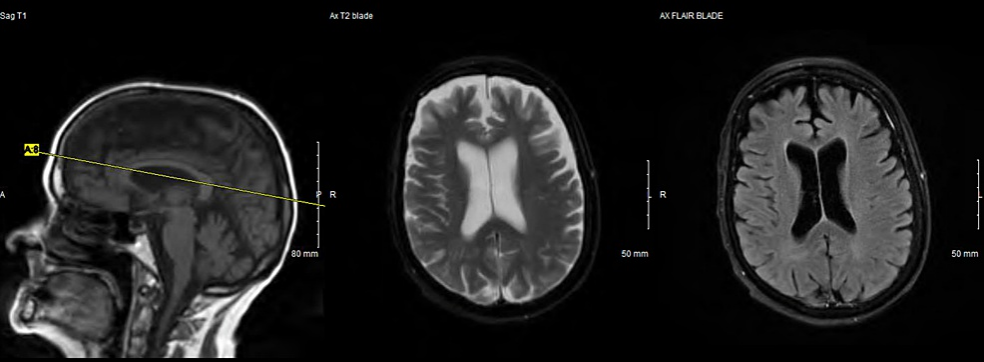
Magnetic resonance imaging with/without contrast of the head 2022 (2)
